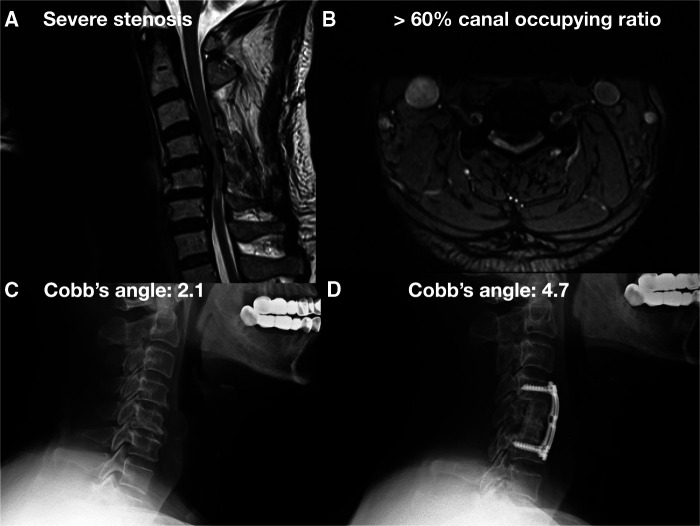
A 63-year-old male with CM due to long-segment massive OPLL over C3-6 underwent C3-6 laminectomy followed by anterior C4-5 and C5-6 microdiscectomy and C5 corpectomy, and C4-6 interbody fusion and internal fixation. (A) Preoperative cervical MRI showed severe cervical stenosis with bulging disc. (B) Preoperative cervical MRI axial view showed >60% canal occupying ratio. (C) Preoperative cervical x-ray showed cervical lordosis about 2.1°. (D) Postoperative cervical x-ray showed cervical lordosis about 4.7°.
